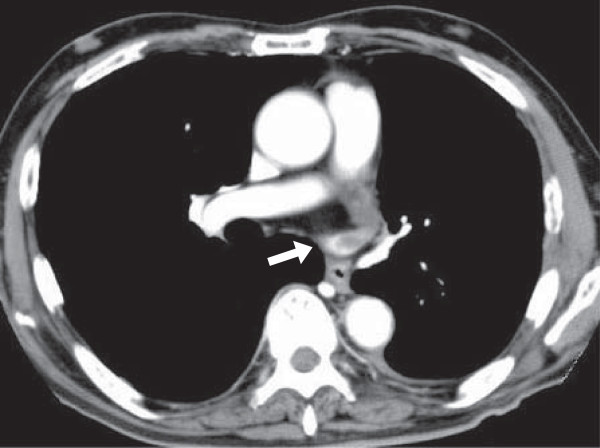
A thrombus in the left superior pulmonary vein stump after left upper lobectomy. Contrast-enhanced CT on a 66 year-old male for follow-up 7 months postoperatively shows a thrombus in the LSPV stump. This thrombus was not detected at that time.
